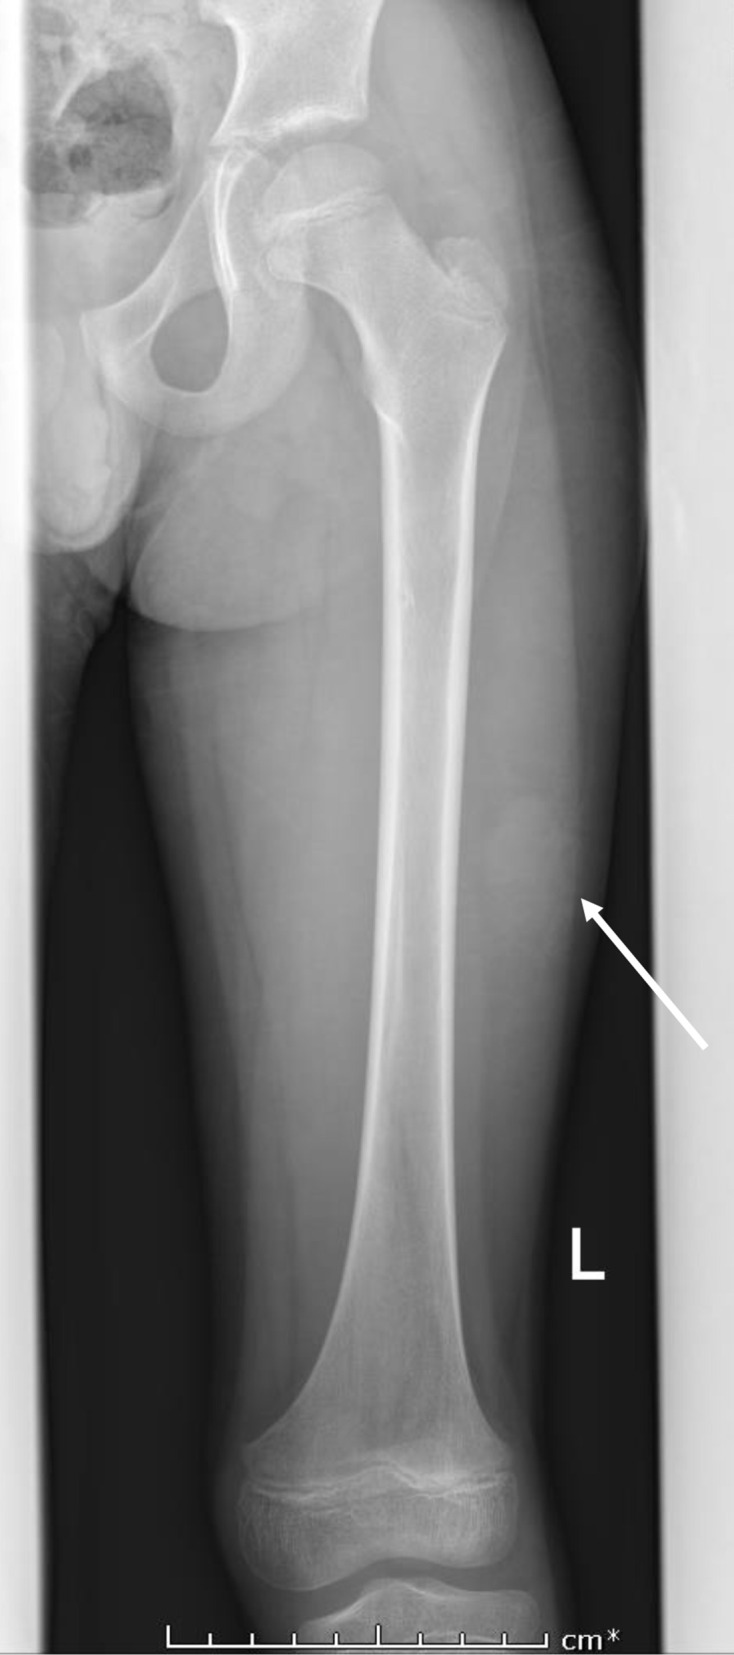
8-year-old male with angiomatoid fibrous histiocytoma. Anteroposterior radiograph of the right femur demonstrating an ovoid soft tissue lesion in the mid-left thigh (arrow).
